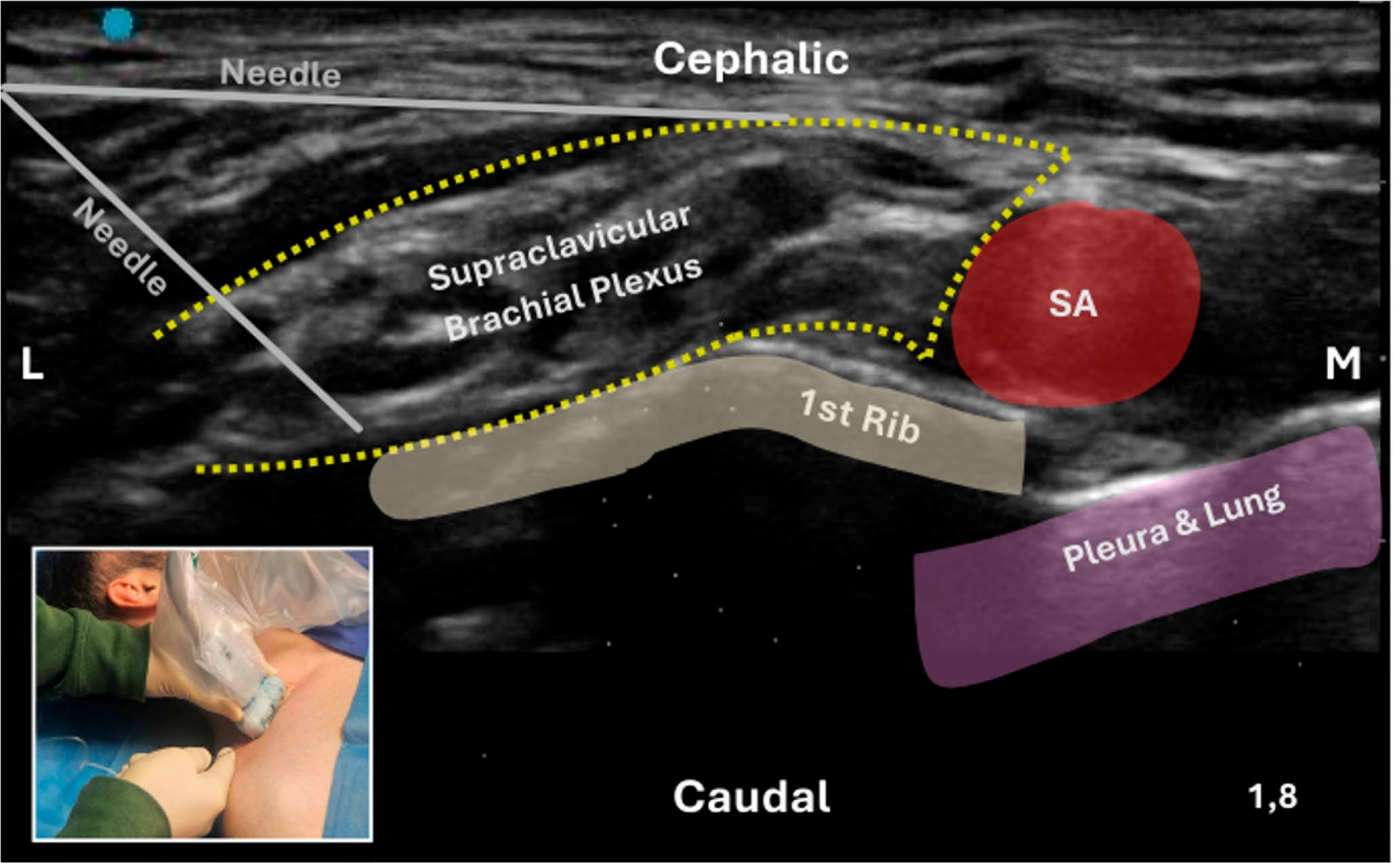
Ultrasound-guided supraclavicular brachial plexus block using a linear transducer with an in-plane lateral-to-medial needle approach (inset). The needle is advanced with one puncture and two minor redirections to ensure optimal spread of local anesthetic around the supraclavicular brachial plexus (yellow dashed line). Key sonoanatomical landmarks include the subclavian artery (SA), the first rib, and the pleura and lung, which should be carefully identified to minimize the risk of pneumothorax. Abbreviations: BP – Brachial Plexus; SA – Subclavian Artery; L – Lateral; M – Medial
